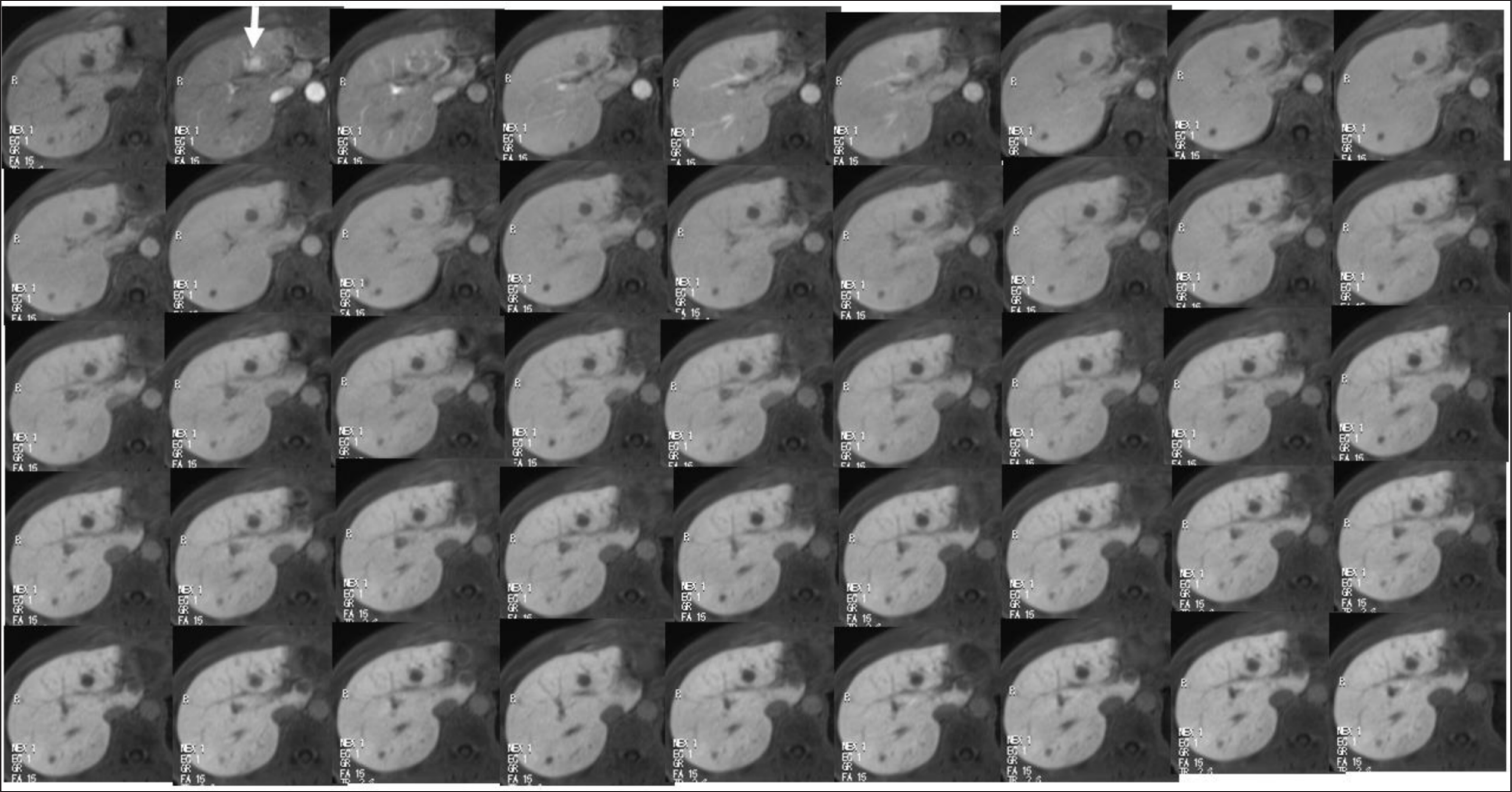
DCE-MRI acquisitions of five images over 30 seconds in each phase were taken pre-contrast, at the hepatic arterial-dominant phase and at 60, 120, 180, 240, 330, 420 510 and 600 seconds post-contrast.
